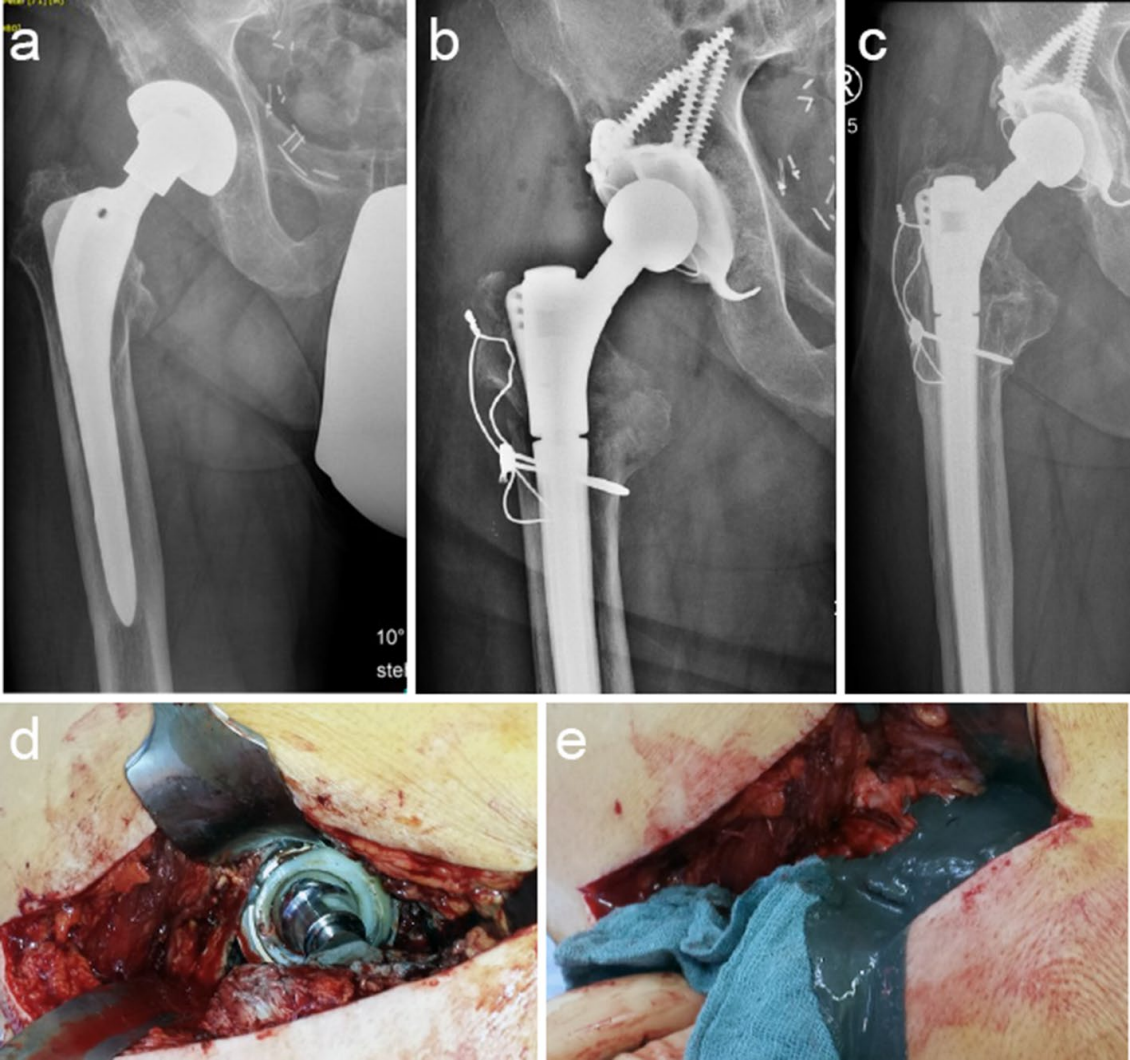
Clinical case of a 73-year-old, male patient presenting with a type 2C defect caused by severe metallosis due to metal-on-metal particle debris. Primary arthroplasty took place 12 years prior to revision; (a) displays the preoperative native radiograph (pelvis ap standing) with osteolytic bone stock at the metaphysis; (b) postoperative native radiograph (pelvis ap standing) with a cementless, modular, femoral revision stem and additional wire cerclages to stabilize the remaining bone stock of the greater trochanter combined with impaction bone grafting; (c) native radiograph (pelvis ap standing) four years after revision arthroplasty displaying a consolidation of the augmented and stabilized femoral metaphysis; (d/e) displays the intraoperative finding of severe metallosis
